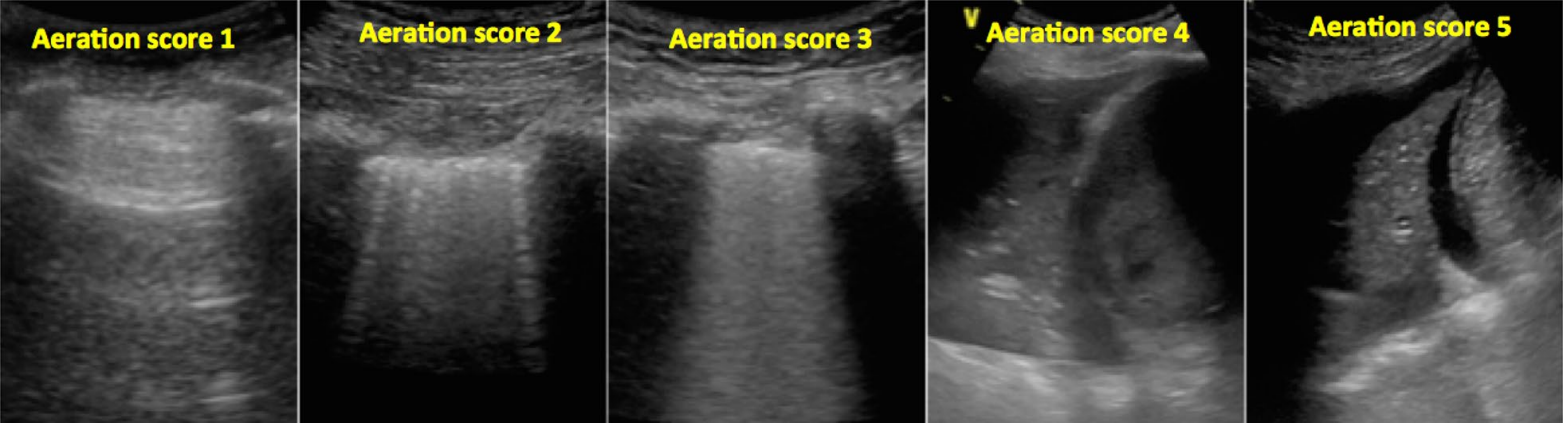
Lung ultrasound aeration scoring. The pattern and the extent of aeration defect is quantified as follows: A normally aerated or fewer than 3 B-lines scored 1; multiple discrete B-lines scored 2; multiple and fused B-lines scored 3; unaerated consolidated lung scored 4; unaerated consolidation with pleural effusion scored 5. Hence, the maximum score for each half was 5 and the maximum score for the posterior zone was 5
